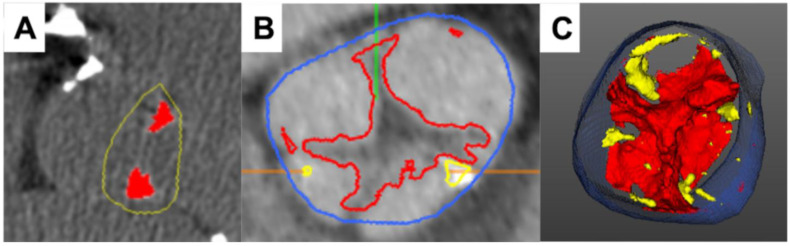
Characterization of aortic valve composition via cardiac computed tomography. Case example of a stenotic aortic valve with fibrotic tissue alterations, which are more pronounced than calcification ((A)—calcium score of 541AU). Computed tomography angiography ((B,C) allows for quantification of both fibrotic (red) and calcific (yellow) tissue components, improving the diagnostic accuracy of the modality (blue marks the aortic lumen; green and orange lines are software markers).
